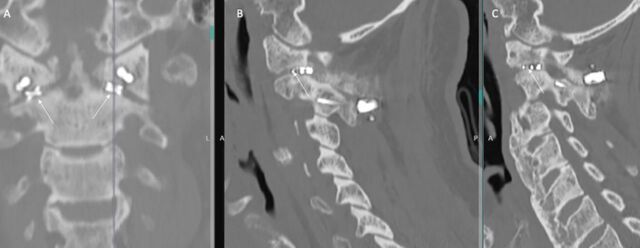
Noncontrast cervical spine computed tomography (A) coronal and (B, C) sagittal sequences centered on the (B) right and (C) left atlantoaxial joints show solid arthrodesis through the intrafacet constructs (arrows).
